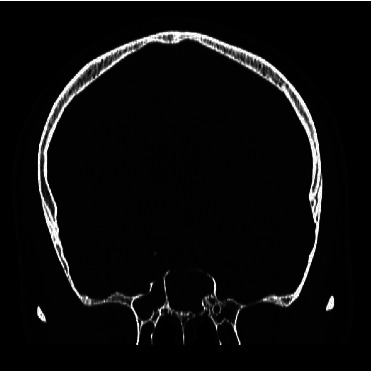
Postoperative coronal CT image demonstrating resolution of pneumocephalus.
